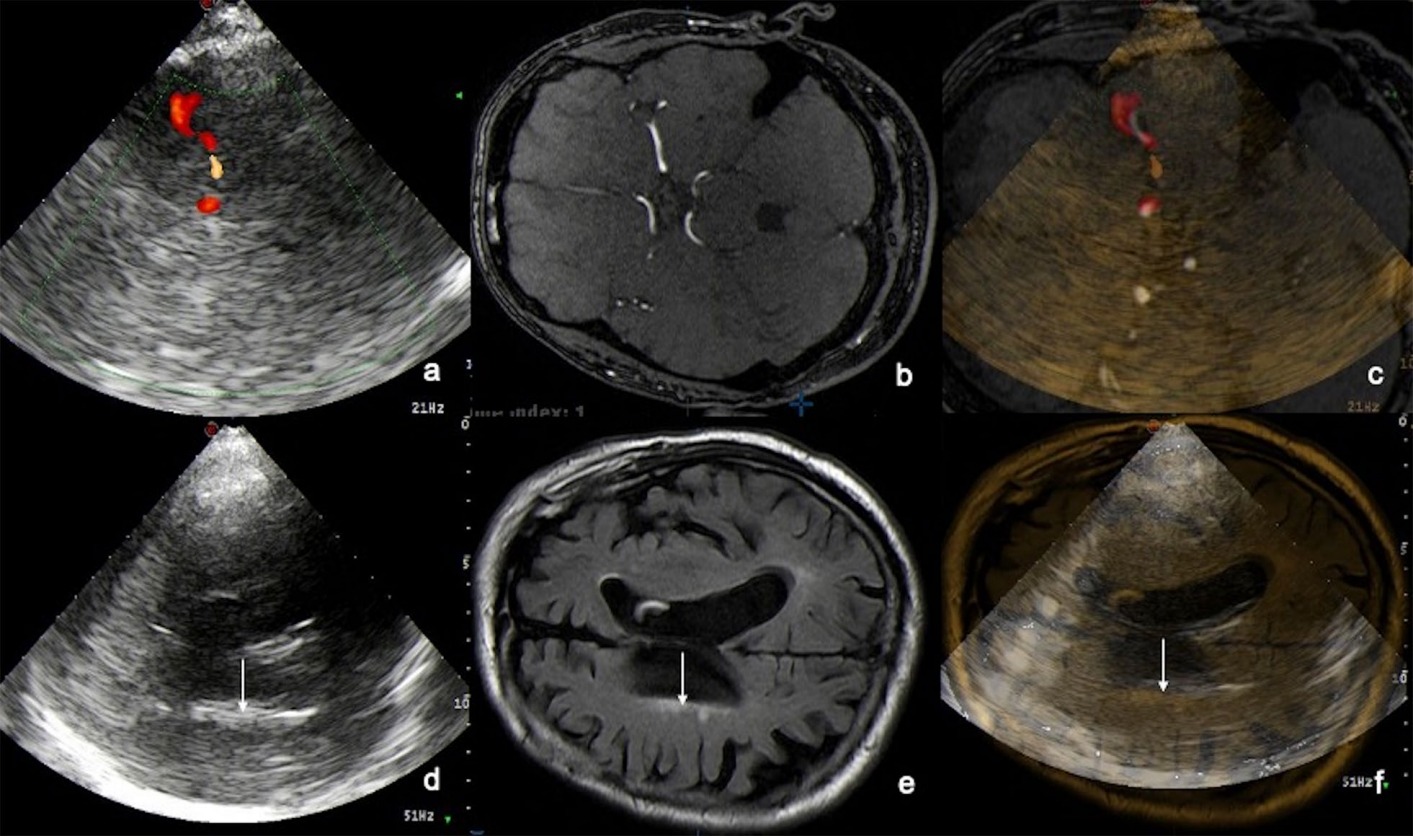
Fusion procedure of ultrasound (US) and MR images. The ipsilateral middle cerebral artery (MCA) is determined as anatomical landmark. After US visualization of the ipsilateral MCA in color-coded duplex ultrasonography (TCCS), axial scanning approach, US images are fused with the corresponding MR images (TOF-sequence) and corrected, if necessary, to match perfectly (a–c). During UFI investigation MR FLAIR images are used for online examination (d–f). a–c: Left: TCCS MCA ipsilateral. Middle: MR TOF angiography. Right: Fused images. d–f: Left: TCS in B-Mode. Middle: cerebral MR FLAIR image, axial plane. Right: fused images US and MRI. White matter lesions (WMLs) II, Anterior horn contralateral. White arrows indicate the WMLs
